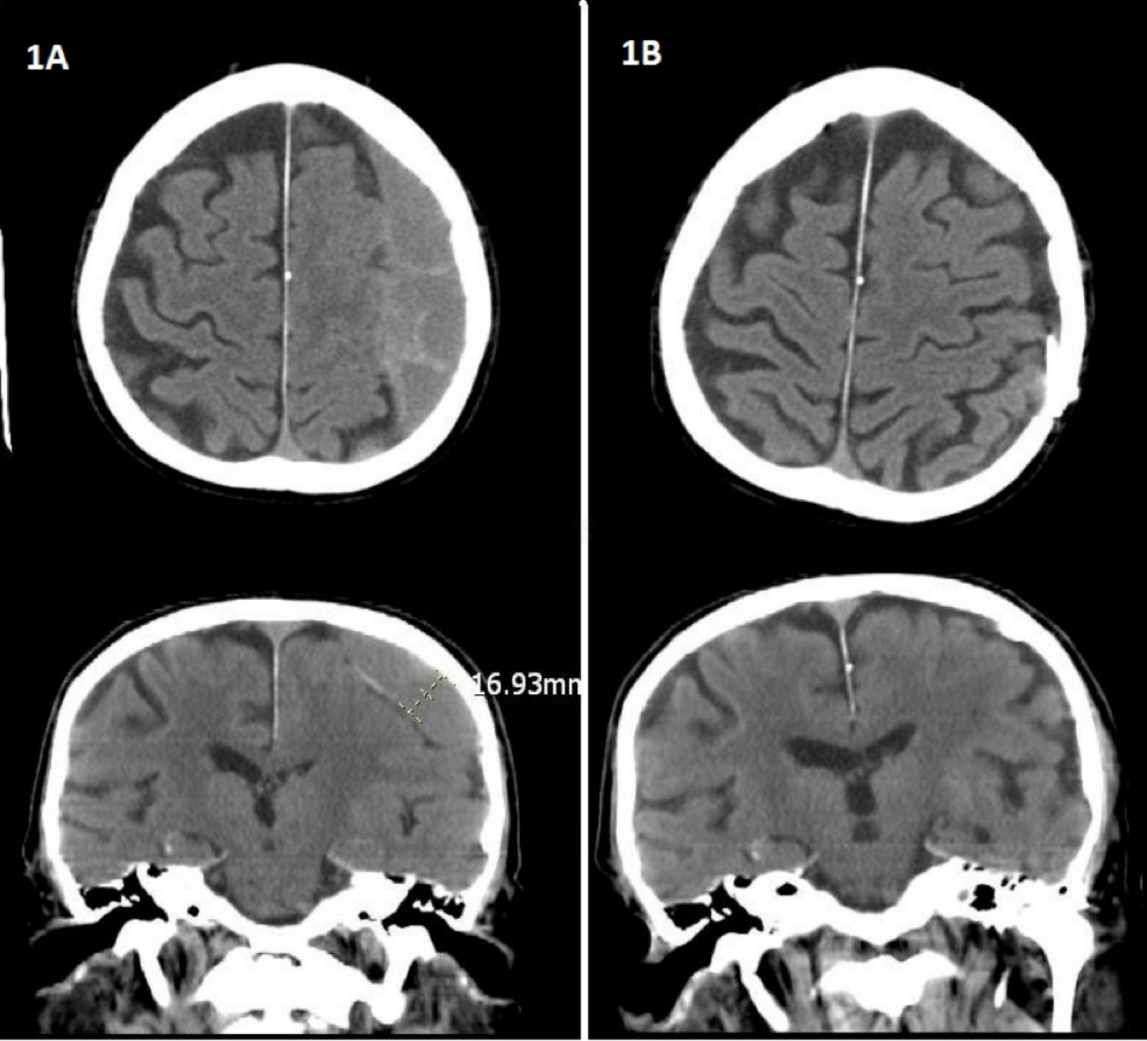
Brain CT Scan Images Figure 1A: Pre-op brain CT scan image demonstrating a large right subacute subdural hematoma causing subfalcine herniation, impending uncal herniation, right to the left shift of 1.0 cm mass effect and ipsilateral ventricles. Figure 1B: Brain CT scan demonstrating status post neurosurgical evacuation of right subdural hematoma. - Burr holes are present at the parietal convexity. Subdural drainage catheter, stable in position. Previously present right subdural air has resolved. No extra-axial fluid collections identified from the level of the foramen of Monro to the convexity. No acute intracranial hemorrhage.
